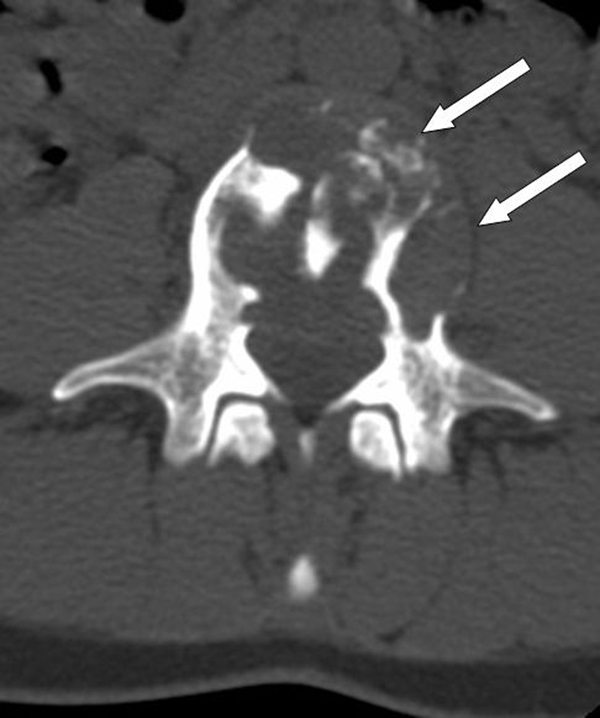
Transversal CT image of the L4 vertebral body, demonstrating remodelling in partially sclerotic bone.
